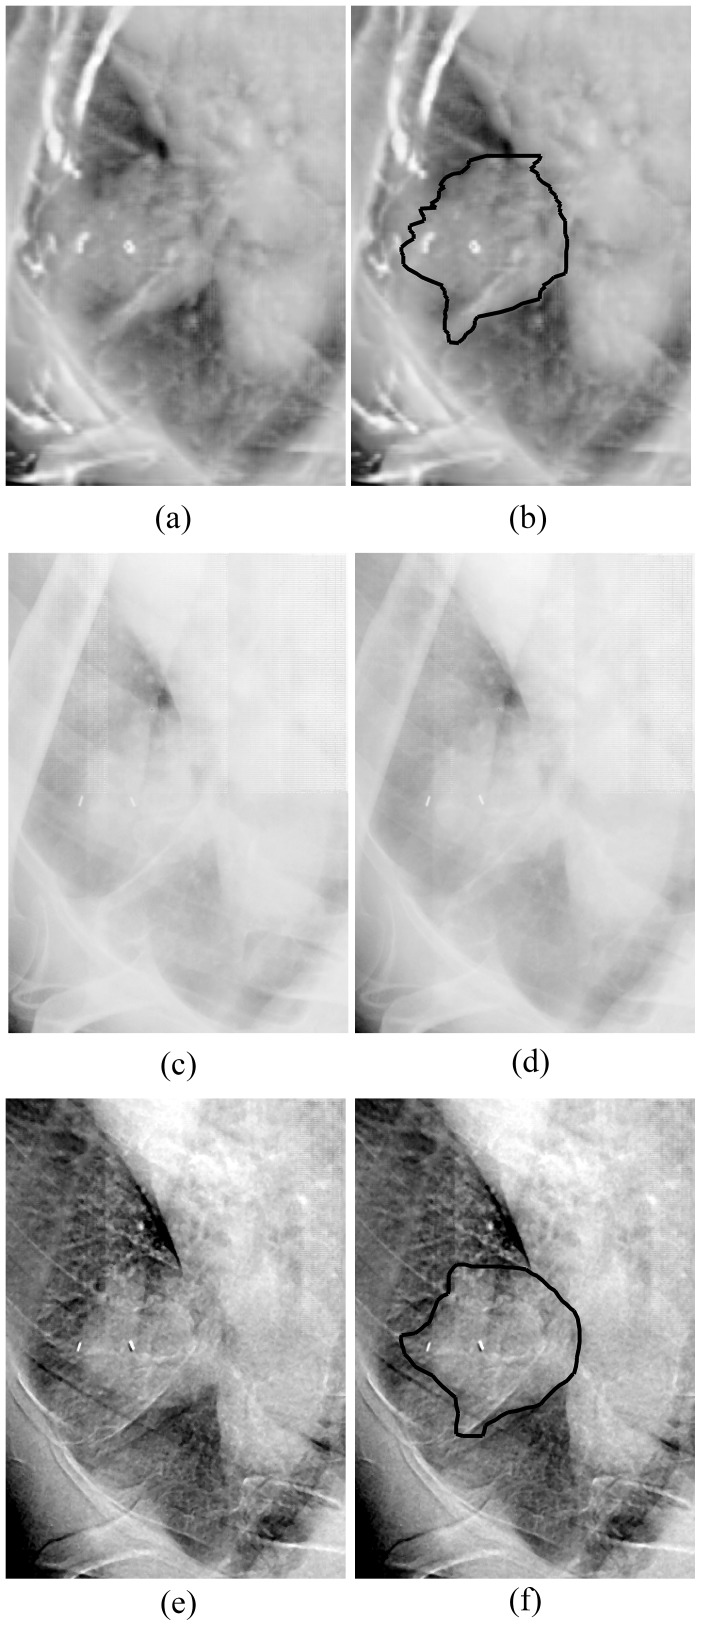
DRRs and radiographs of a patient. (a) DRR of a patient, (b) DRR with the projected GTV contour, (c) radiograph at LE, (d) radiograph at HE, (e) bone-canceled image by pairing two original radiographs, and (f) bone-canceled image with the GTV contour automatically segmented.
